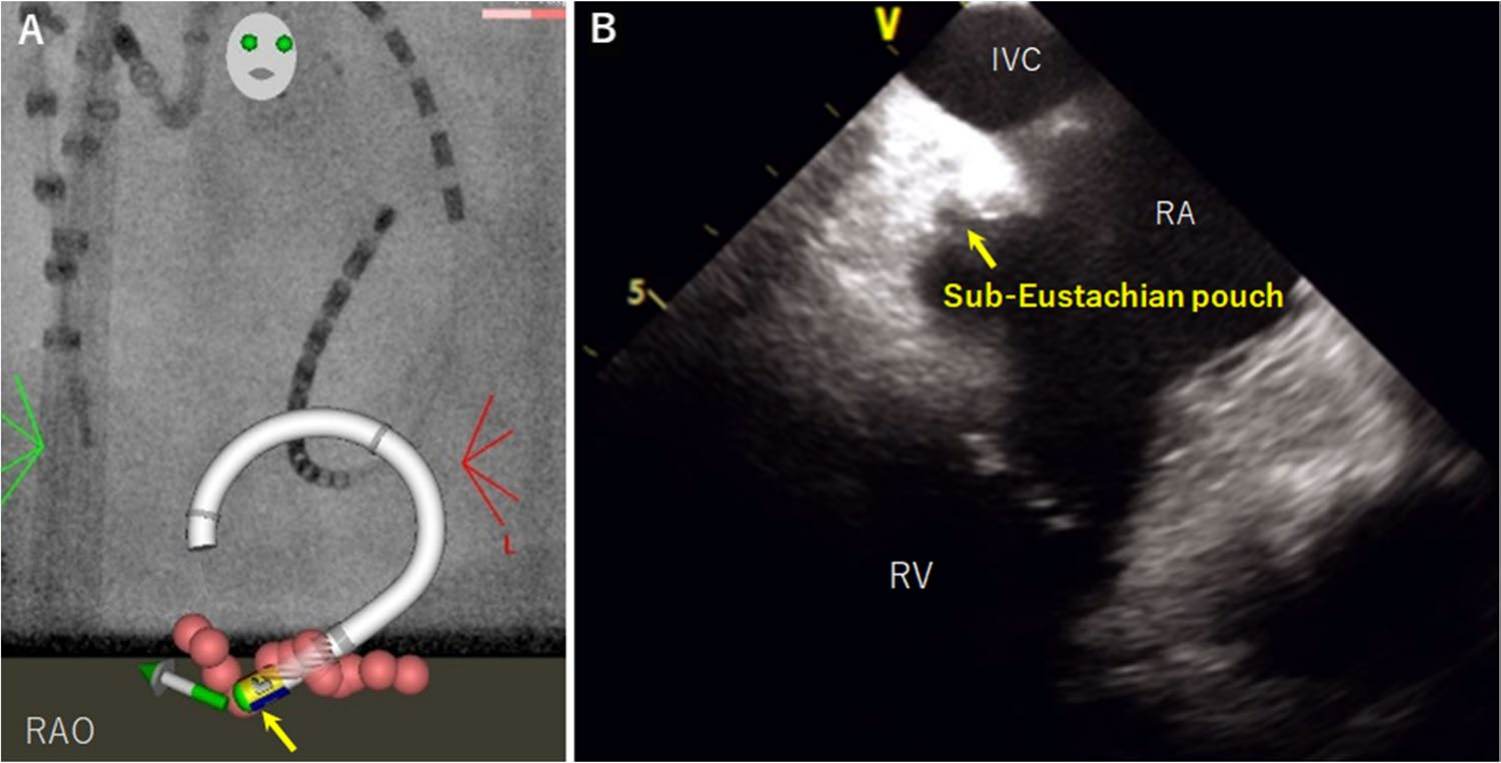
Representative case requiring additional ablation because of a sub-Eustachian pouch. A Right anterior oblique (RAO) projection of CARTO UNIVU in a patient where the first-pass conduction block was not achieved. Additional radiofrequency application with an ablation catheter arched over the Eustachian ridge achieved a bidirectional conduction block. B Intracardiac echocardiography revealed a sub-Eustachian pouch where additional radiofrequency was applied (yellow arrow). IVC, inferior vena cava; RA, right atrium; RV, right ventricle
